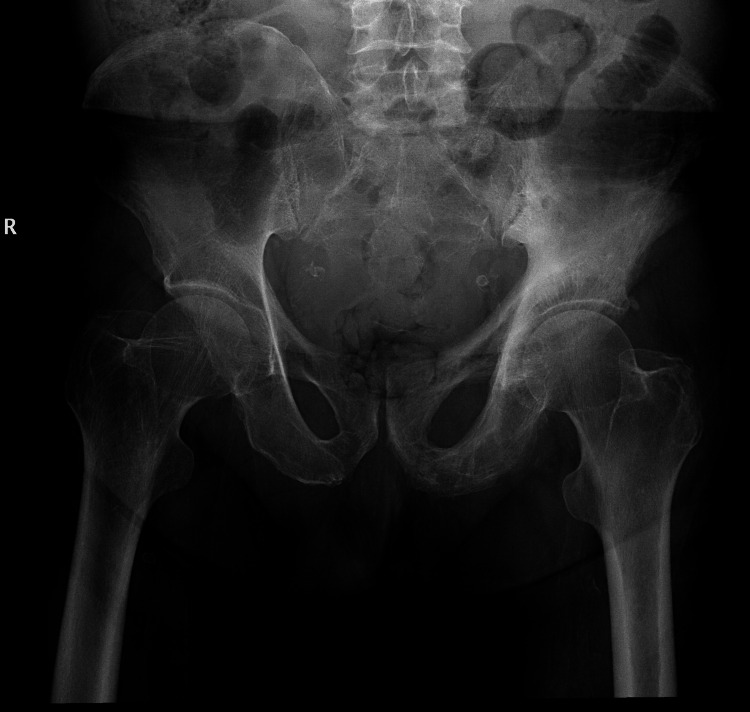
A plain anterior-posterior (AP) radiograph of the pelvis demonstrated coarse bone trabeculation, sclerosis, and bone expansion of the left hemipelvis, with thickening of the left iliopectineal and ilioischial lines
